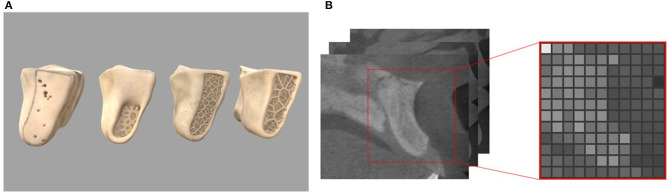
Four classes of the alveolar bone and read gray values from CT slices (A) geometric models of different bone types (B) CTCT slices and corresponding volume slice.
